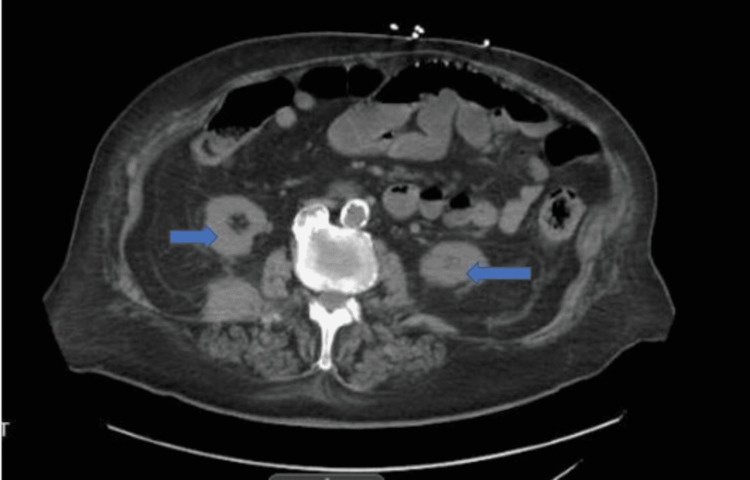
CECT of the abdomen (transverse section) with thickened intestines suggestive of colitis (arrows) CECT: contrast-enhanced computed tomography
